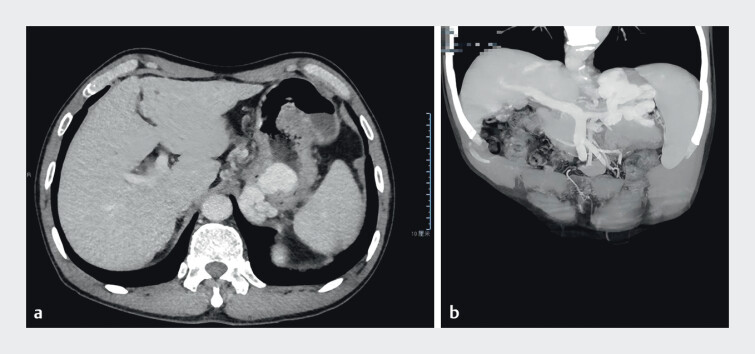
Preoperative abdominal and pelvic CTA revealed a massive variceal mass in the gastric fundus, which was connected to extramural vessels, indicating the presence of GRS.
